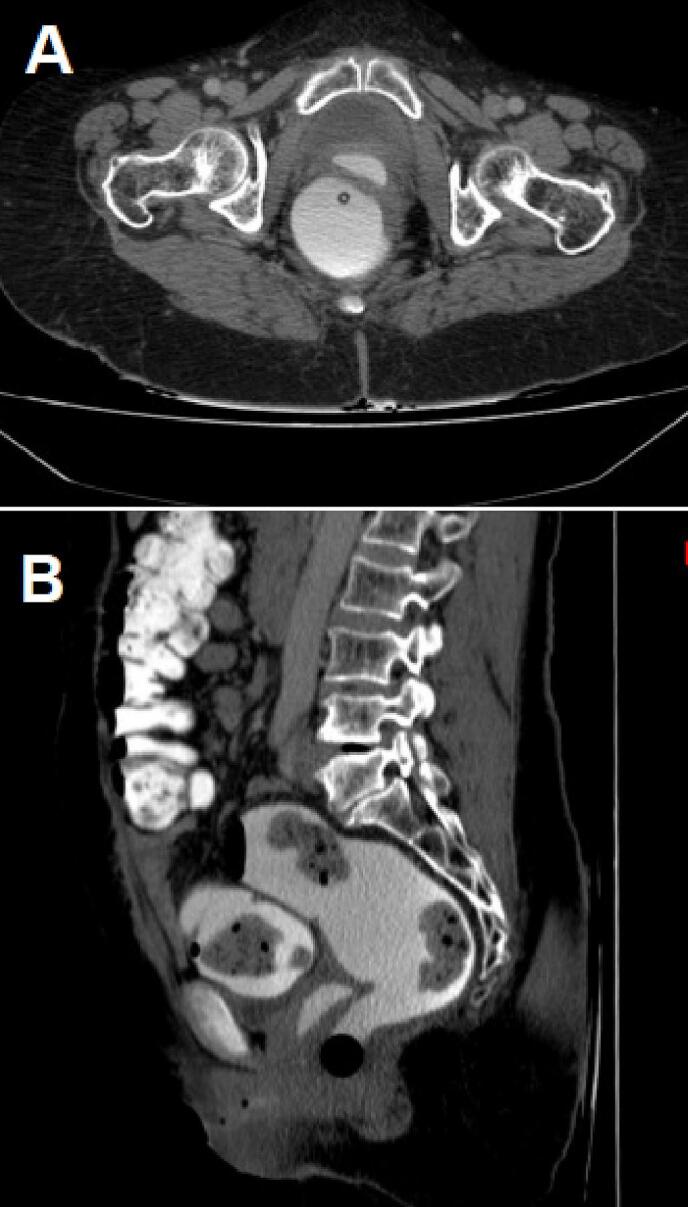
(A and B). CT scan images after opacification by vaginal route showing the passage of the contrast product at the vaginal level revealing a rectovaginal fistula.
